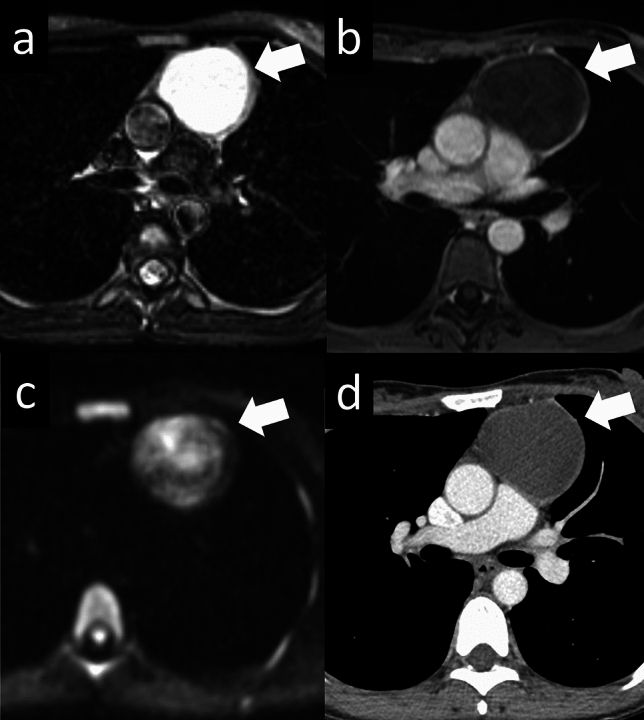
Mature cystic teratoma. A 35-year-old woman with mature cystic teratoma. a Fat-suppressed T2WI shows a mass lesion in the anterior mediastinum with high signal intensity (arrow). b Contrast-enhanced T1WI shows enhancement of the capsule (arrow). c DWI shows heterogeneous high signal intensity. d CT shows a 6.0 cm cystic mass (arrow). T2WI, T2-weighted imaging; T1WI, T1-weighted imaging; DWI, diffusion-weighted imaging; CT, computed tomography
